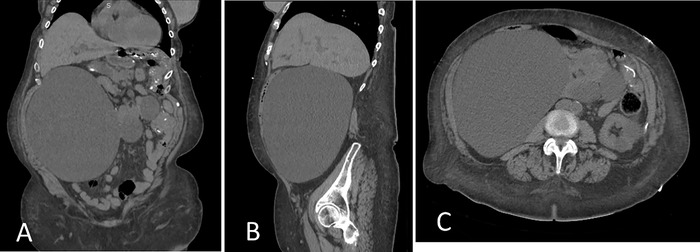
Computed tomography of the abdomen revealing a massive simple cyst on (A) coronal, (B) sagittal, and (C) axial views.
